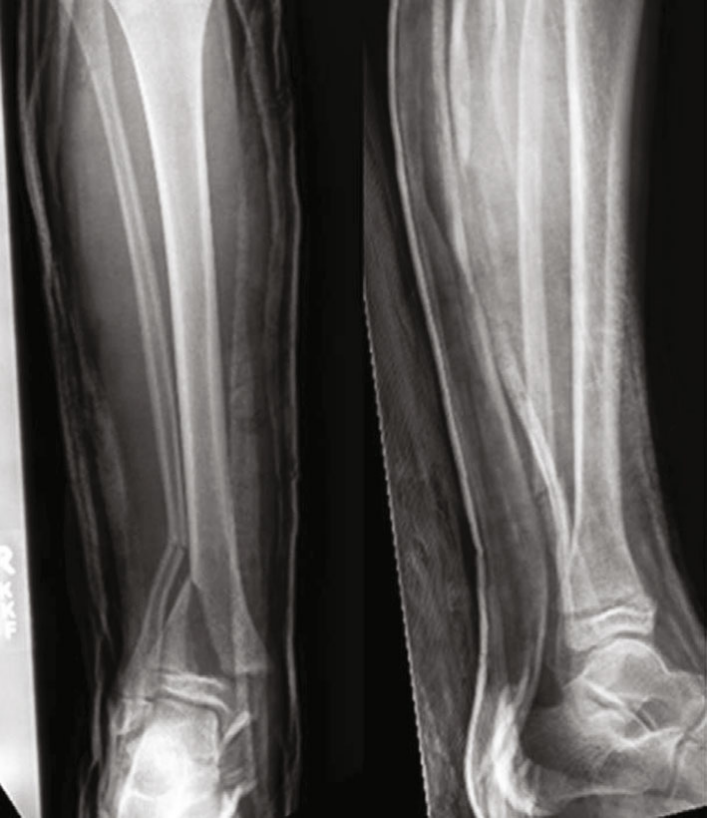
Anteroposterior and lateral radiographs of the ankle following attempt of closed redcution.
